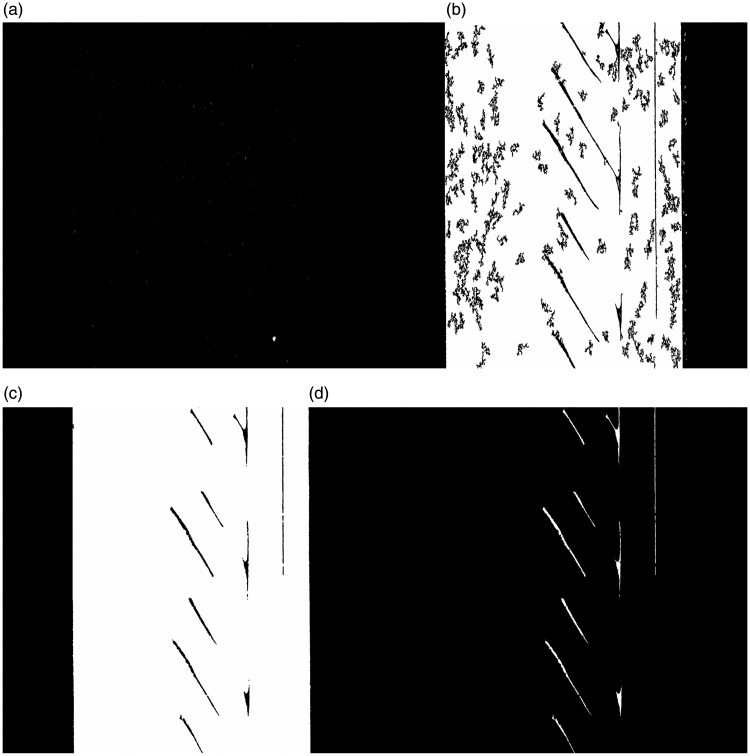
Pores identification steps for copper PLA sample: (a) µ-CT image, (b) filtered and despeckled, (c) large despeckled and closed (d) inversed image highlighting air gaps.
